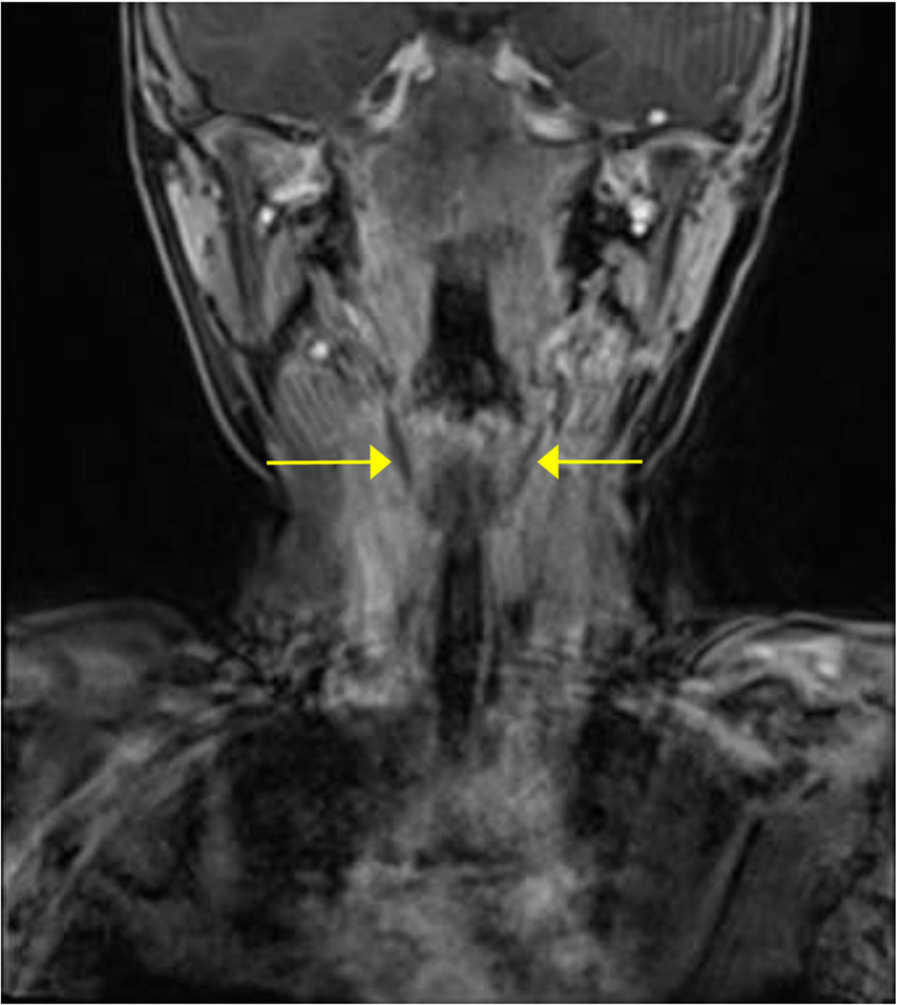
Coronal MRI identifying bilateral tracts from the piriform sinus to the thyroid gland
